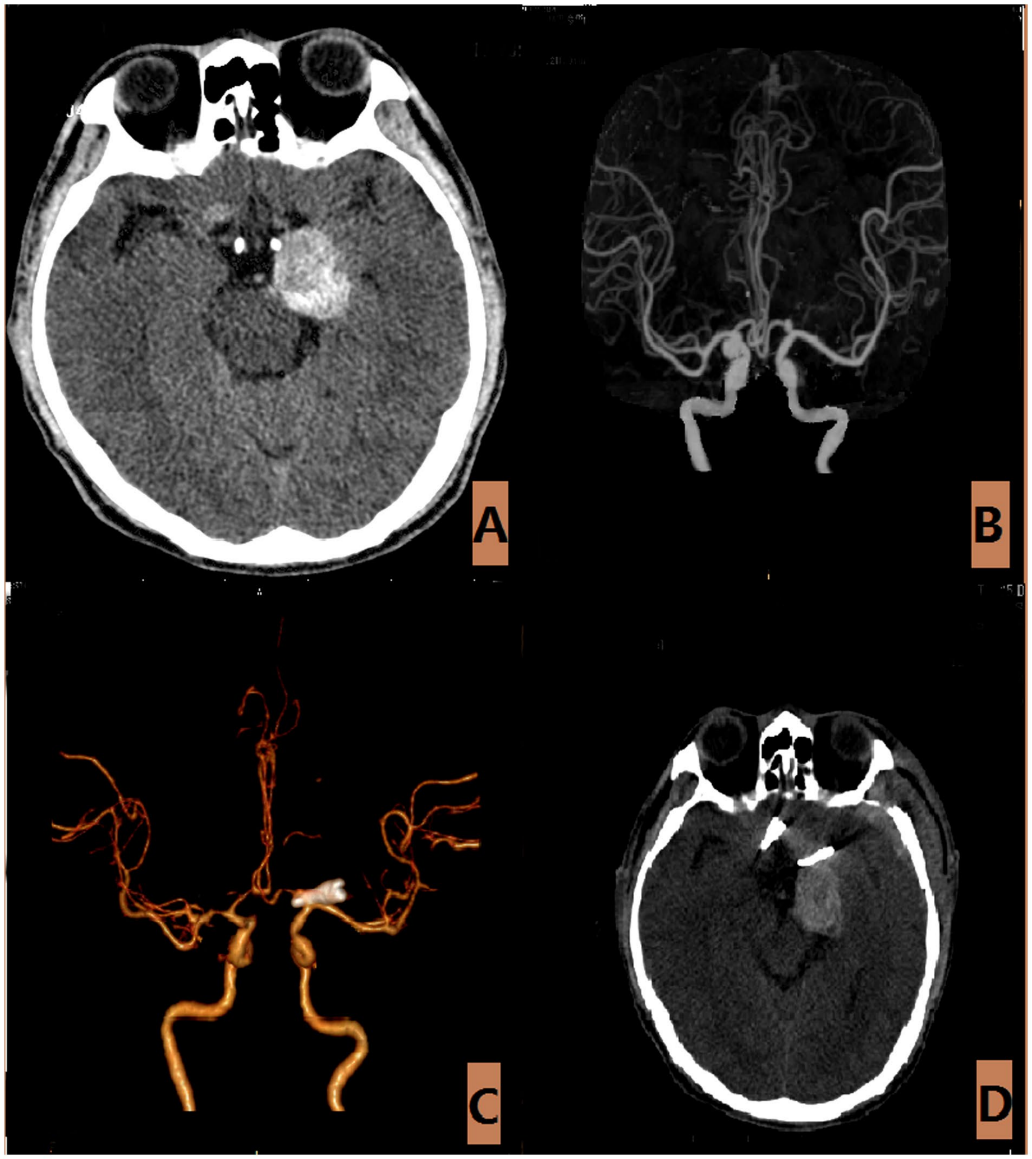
The aneurysm is of the largest variety (Type-V). (A) The CT scan of a 60-year-old male with an unruptured large aneurysm, revealed occupying lesions throughout the brain. (B) The CTA revealed that the aneurysm measures approximately 2.7 × 6.0 millimeters. (C,D) Postoperative CTA and CT scan.
